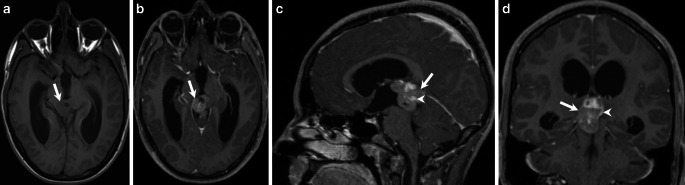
On nonenhanced axial T1-weighted images (a) the lesion (*arrow*) is isointense when compared to the brain parenchyma and does not show any signs of infiltration of the surrounding tissue. After administration of gadolinium the lesion (b–d, *arrow*) shows marked enhancement on axial (b), sagittal (c), and coronal (d) T1-weighted images. Note the deep cerebral veins entrapped within the tumor matrix (c, *arrowhead*) and the delicate capsule surrounding the lesion (d, *arrowhead*)
